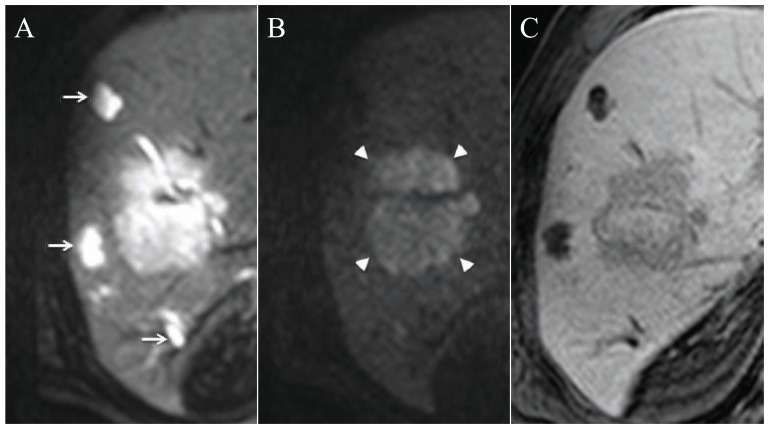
A patient with a large liver metastasis (from colorectal cancer) and three cysts. On DWI (A, B) the cysts (arrows) have a benign
